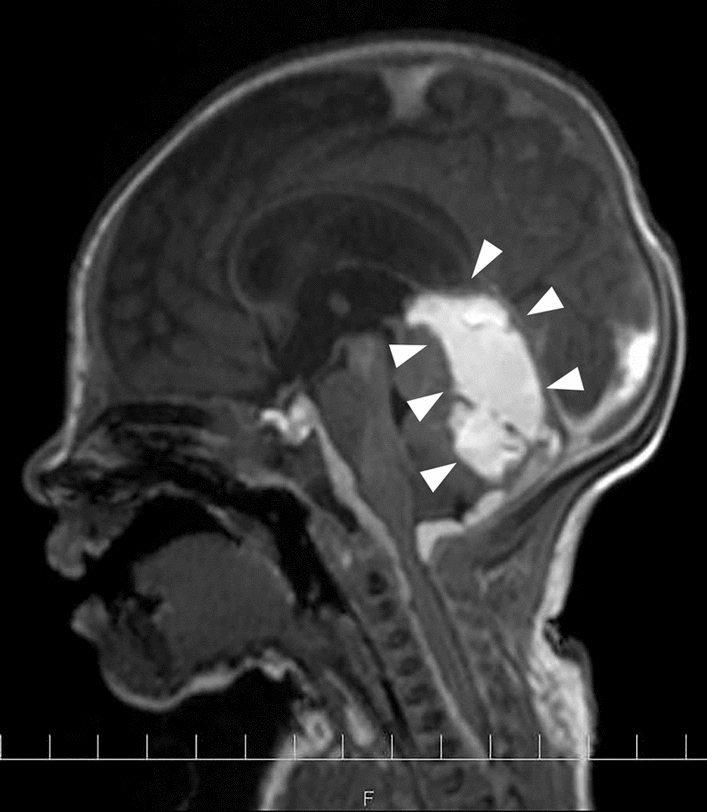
Cranial magnetic resonance imaging (sagittal view) on day 13. Infratentorial and cerebellar hemorrhages observed (arrowheads)
